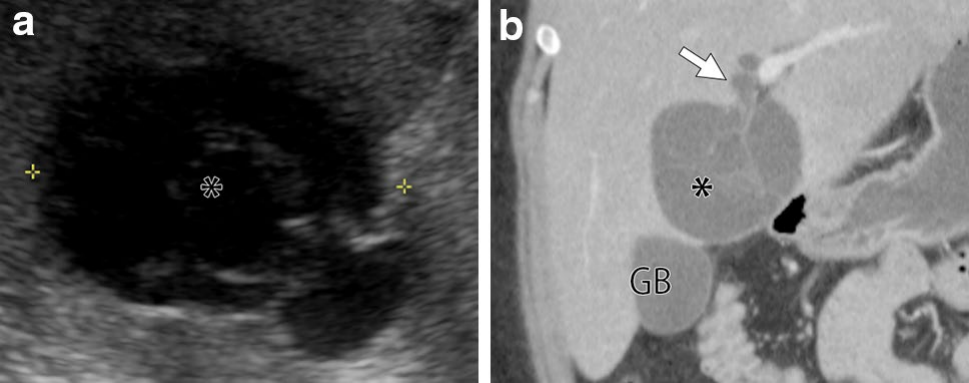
A 23-year-old woman presented with abdominal pain. She had a multilocular cystic mass 75 mm in diameter in the segment IV of the left lobe of the liver on US (a, asterisk) and contrast-enhanced CT (b, coronal image, asterisk). Arrows indicated the tumoral prolapse to the bile duct. Both readers could not tell the biliary prolapse on US, but could tell on CT. Abbreviation: GB, gallbladder
